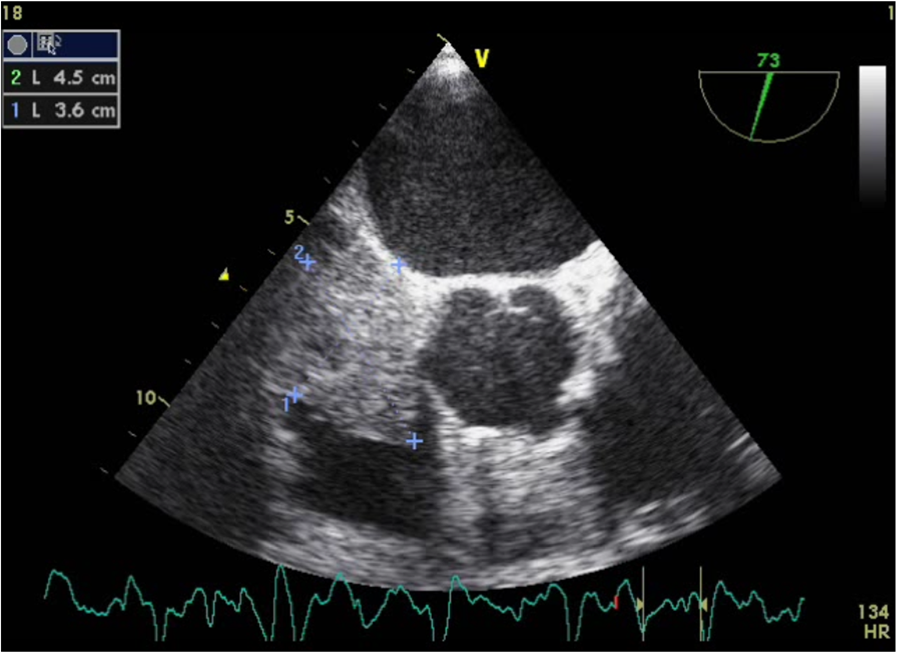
Transesophageal echocardiography demonstrating a myxoma of 5.6 × 3.6 cm rising from the right atrium.
